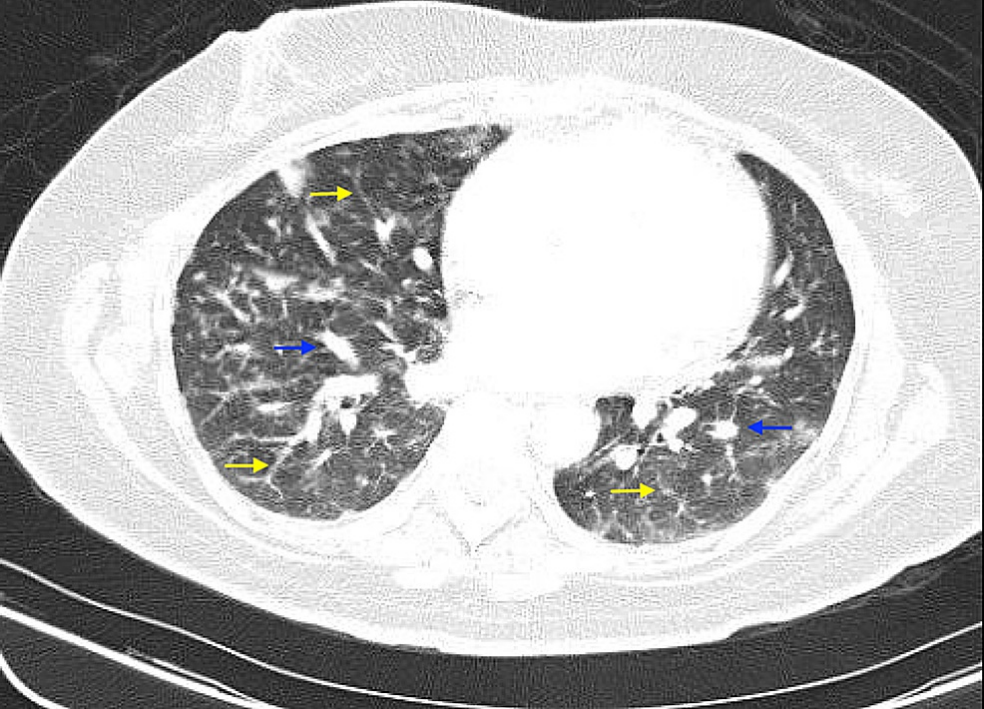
Computed tomography scan with contrast showing bilateral reticulonodular opacities (yellow arrows) and multiple pulmonary nodules (blue arrows).
